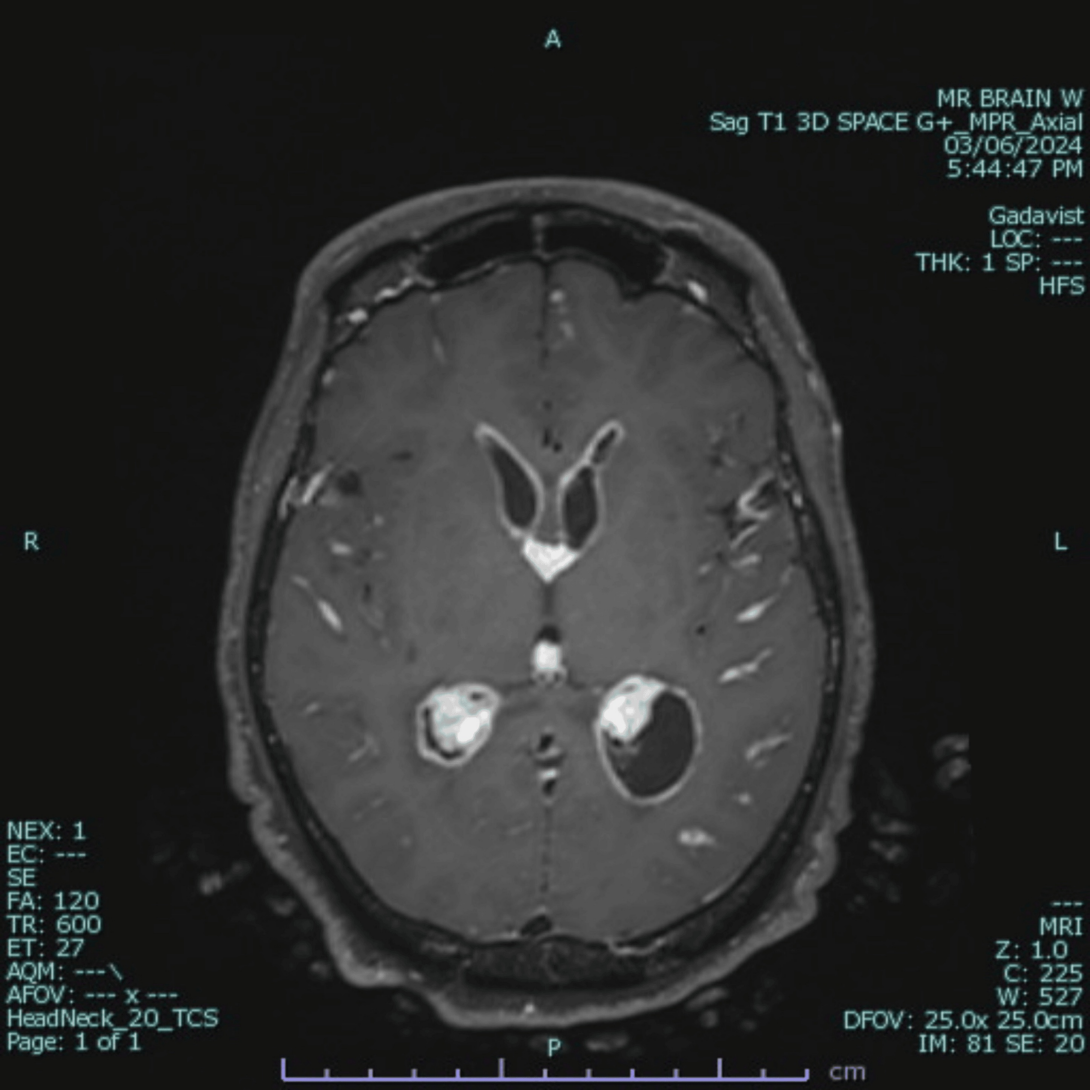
Axial T1-weighted post-contrast MRI of the brain demonstrating leptomeningeal enhancement of cranial vessels, ventricles, pia mater, and the choroid plexus
